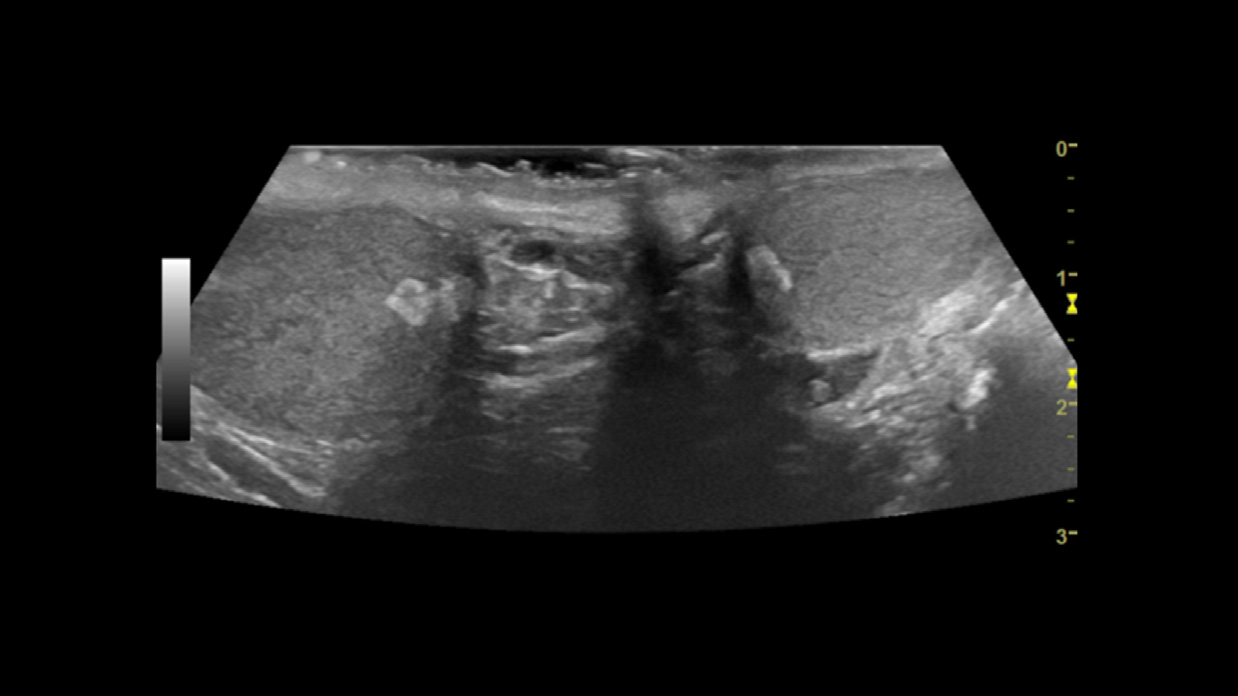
Follow up transverse testicular ultrasound in 2 mo displays unchanged size of the small mixed echogenicity masses in the bilateral testes.
